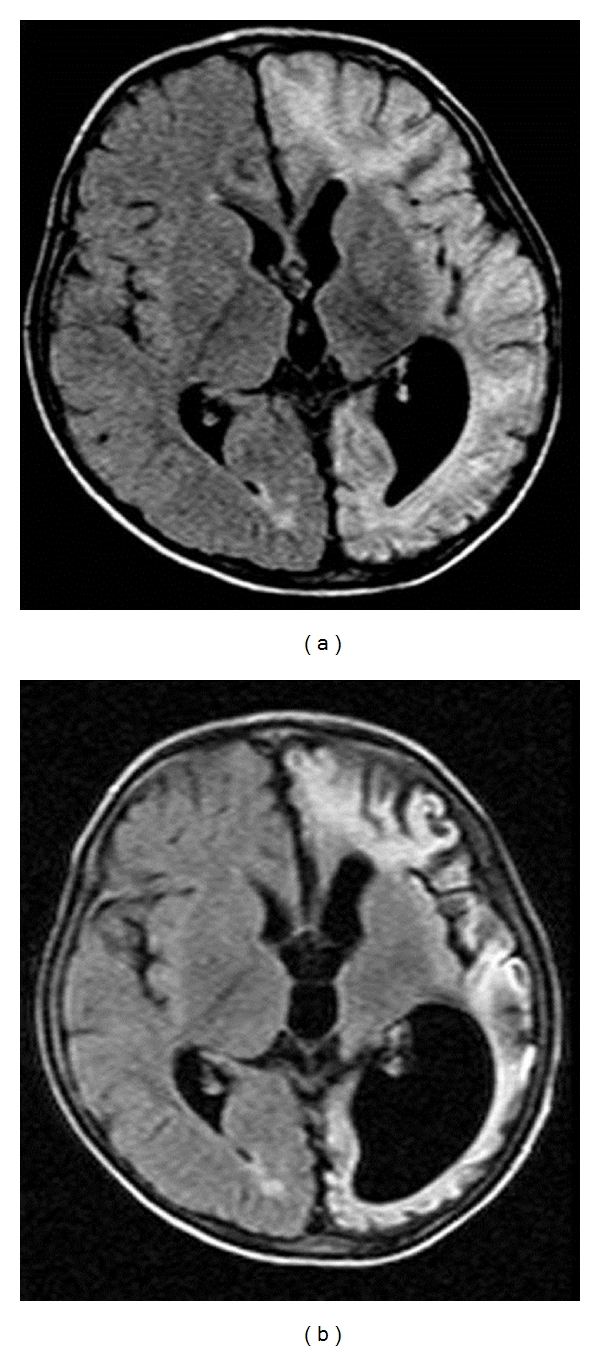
T2W Axial flair MRI images of a patient with Rasmussen's encephlitis taken six months apart showing progression of disease.
